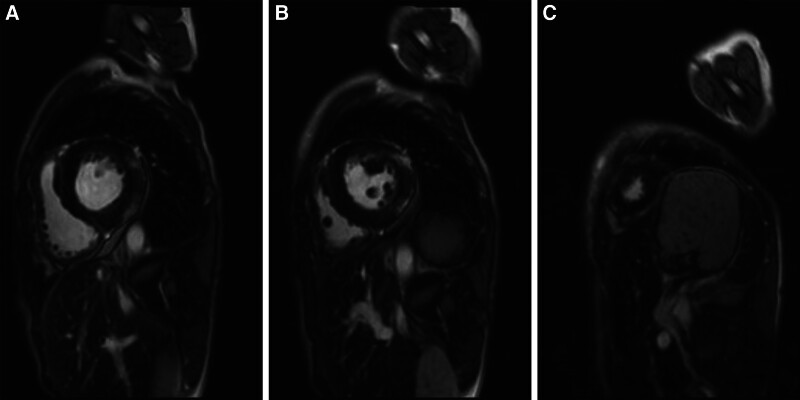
Delayed gadolinium enhancement of the cardiac magnetic resonance images of Patient 1. (A) Left ventricular (LV) base, (B) mid LV, and (C) LV apex.
